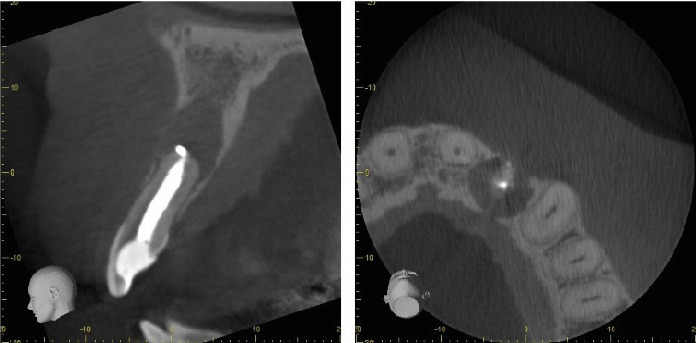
Clinical features of Case Report 1. Preoperative CBCT snapshot of Tooth #22.
